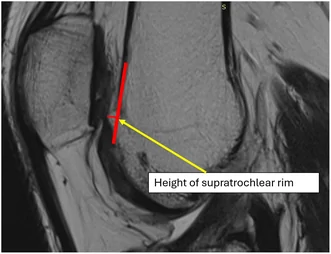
Image of the left MRI sagittal slice with a measurement of the supratrochlear rim height. The measurement is performed on a T1‐weighted sagittal MRI slice where the deepest point of the trochlear groove is visible. First, a reference line is drawn along the anterior femoral cortex. Then, from the highest point of the supratrochlear rim, a perpendicular line is extended to meet this reference line. The vertical distance between the rim's apex and the cortex line represents the rim height. These measurements are crucial for surgical planning. The patient will be considered for rimplasty if the height exceeds 3 mm. This MRI slice shows the right knee of a young male with anterior knee pain, pain during squatting, and a patellar chondral defect. Rehabilitation was unsuccessful, and the patient was subsequently prepared for surgery. (MRI, magnetic resonance imaging.)
